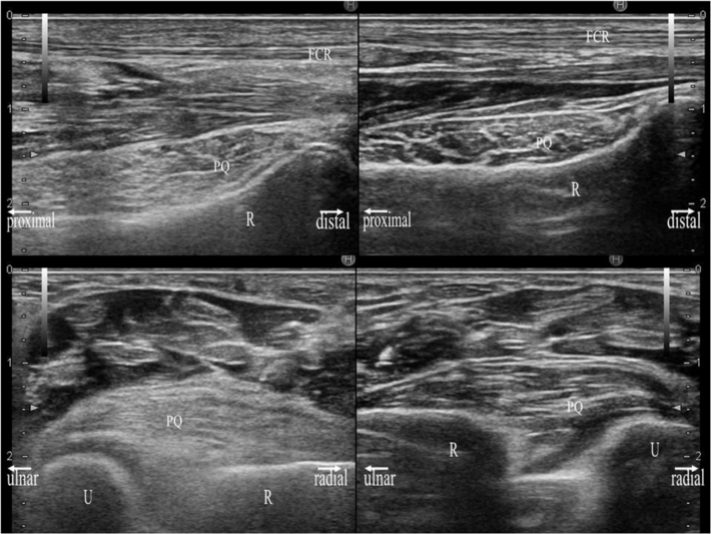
Sonographic images of a 71-year-old man with a diagnosis of distal radius fracture of the left hand. Sonographic images in the longitudinal (upper image) and transverse (lower image) planes were obtained using a 17-MHz transducer. Each image shows a side-by-side comparison of the right hand (right image) and left hand (left image). The PQ of the left hand is prominently thickened with hyperechogenicity. The PQ shows a convex appearance in either hand.
